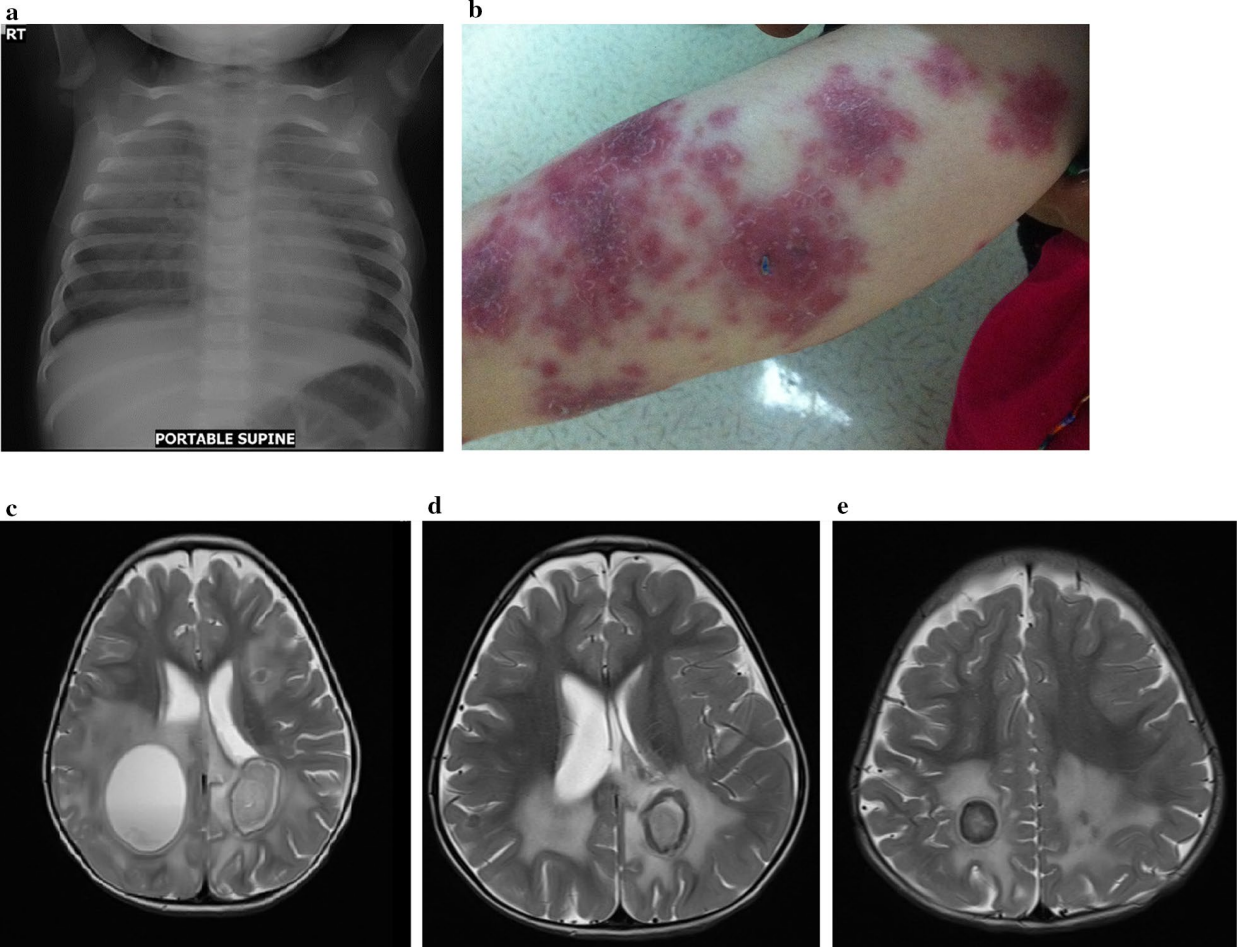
Case 2.  a (age 9 months): chest x-ray at her initial presentation showing bilateral pneumonia. b
